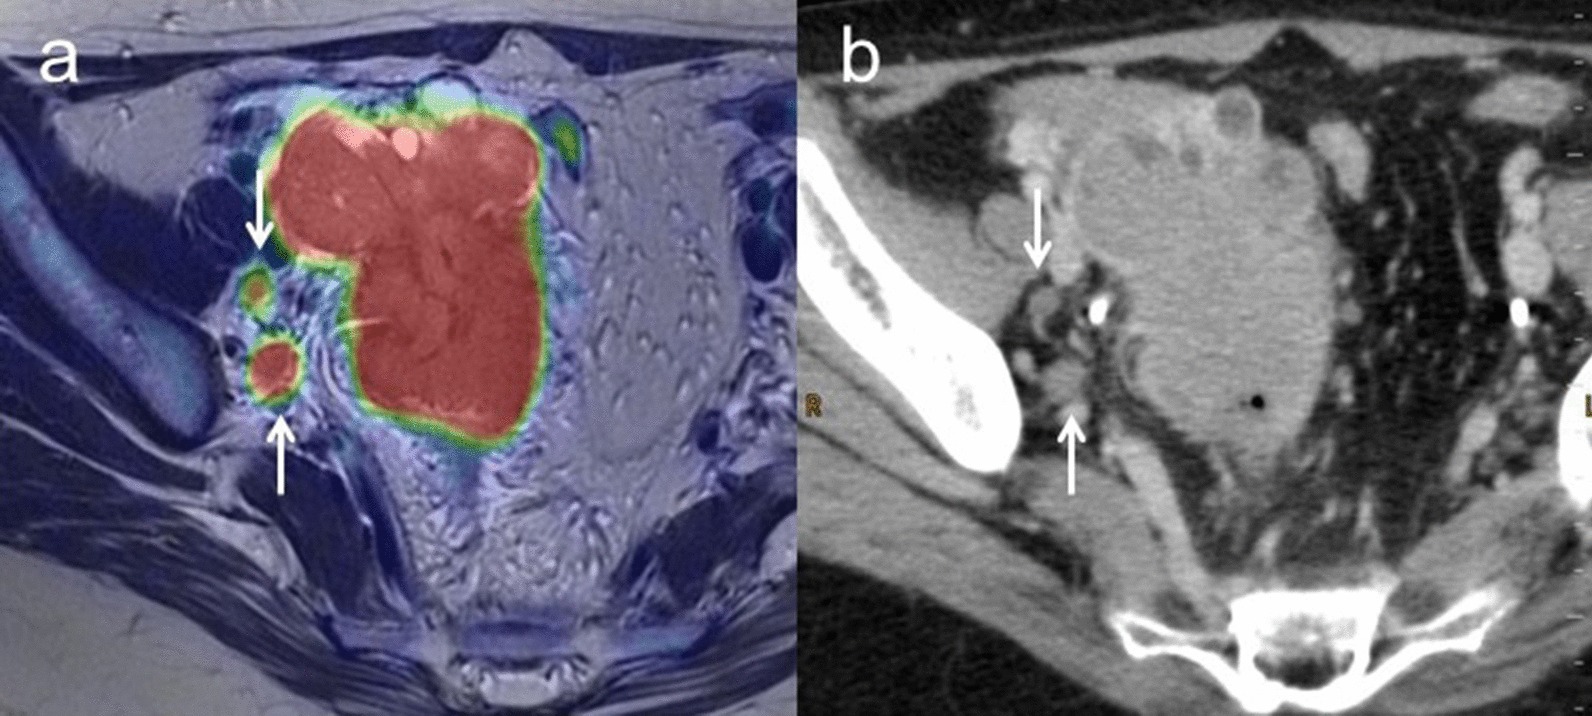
a A 50-year-old woman with pathologically confirmed ovarian cancer. Axial T2-weighted PET/MR image shows right pelvic lymph nodes with FDG uptake (arrow). b Contrast-enhanced CT shows right pelvic lymph nodes less than 1 cm in short-axis diameter without enhancement (arrow). After NAC, these lymph nodes are decreased in size and SUV, suggesting these nodes as a sign of malignancy (N1)
